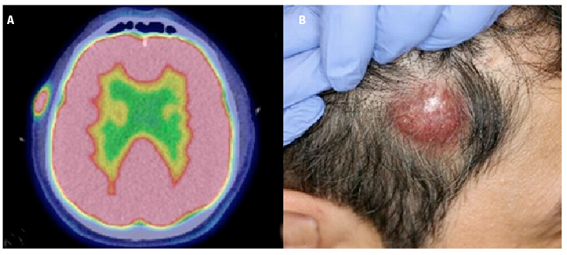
Clinical findings: (A) PET/CT taken at the nearby hospital. A skin lesion with an accumulation is seen in the right temporal region away from the skull. (B) Clinical findings at the first visit: a smooth, soft, and mobile red mass measuring 2.5 × 2 cm with no surrounding purpura.
